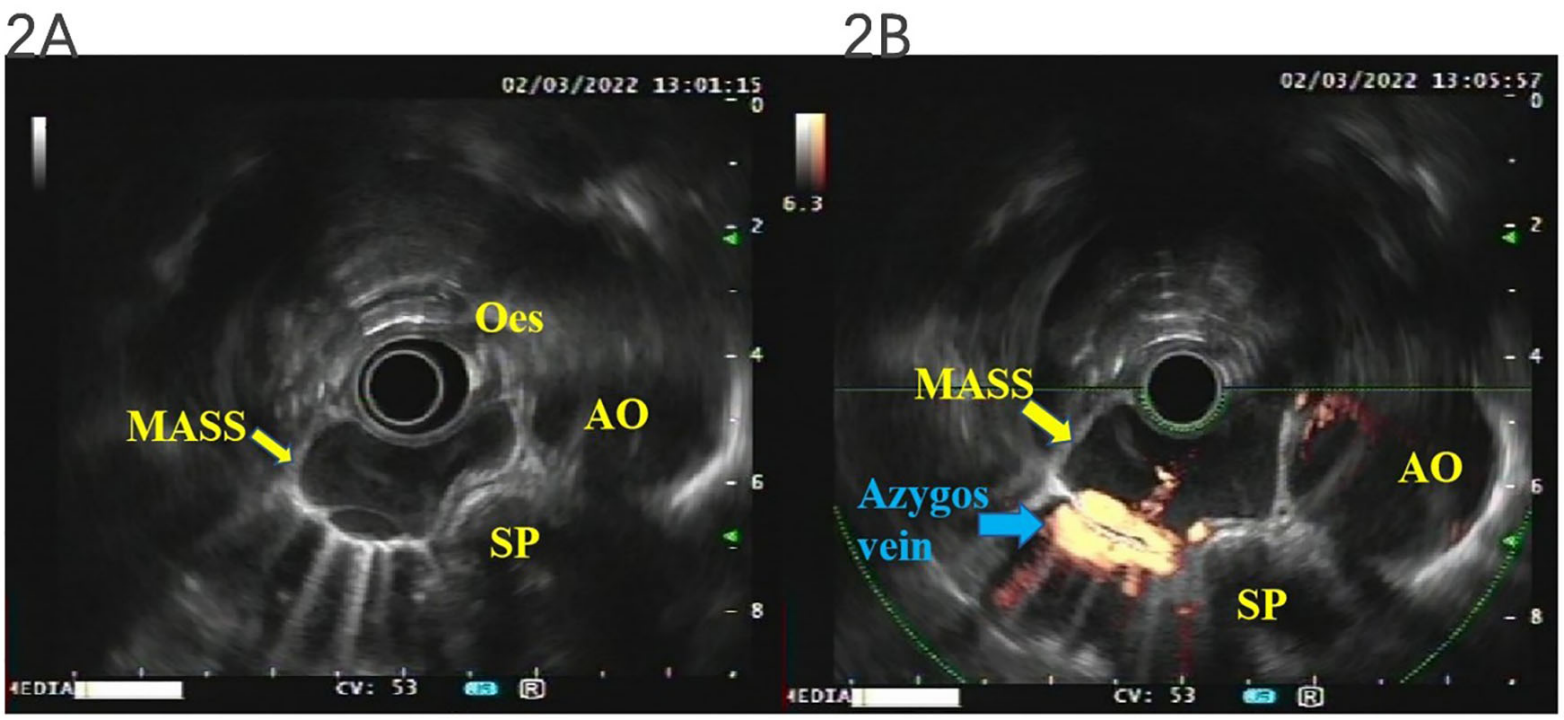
EUS. The mass was not a swollen lymph node but an idiopathic cystic tumor with no internal blood flow signal, and there was no blood flow communication between the posterior azygos vein and the thoracic aorta (A, B). EUS, endoscopic ultrasonography.
